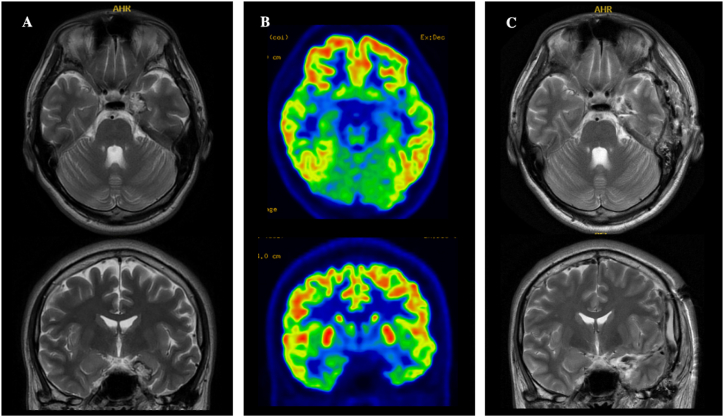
Axial- and coronal-view MRI and FDG-PET. T2-weighted MRI reveals a popcorn lesion with central hyperintensity and a peripheral hypointense rim in the left amygdala (A). This finding is compatible with cavernous malformation. FDG-PET shows slight glucose hypometabolism in the left mesial temporal (B), consistent with the lesion on MRI. MRI at 3 days after the removal surgery shows complete resection of cavernous malformation (C).
